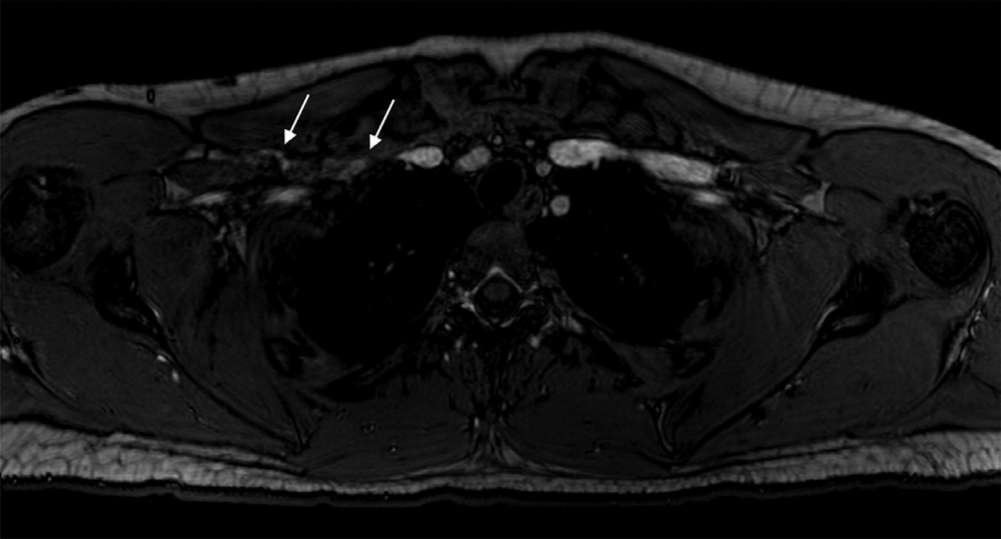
Filling defects in the right subclavian vein (white arrows) with arms in the downward position, consistent with the patient's duplex ultrasound findings of a right-sided deep vein thrombosis.
